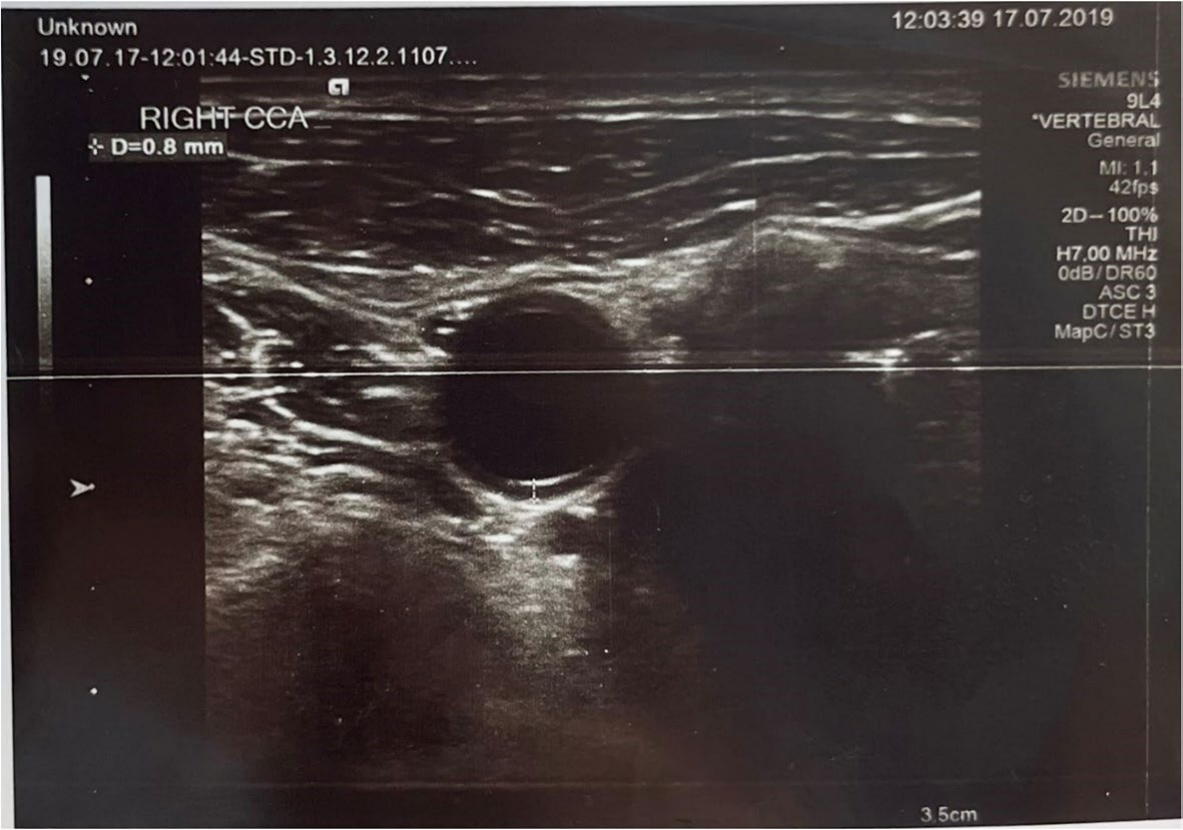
Measurement of right common carotid artery (CCA) in COPD diagnosed patient by ultrasound
